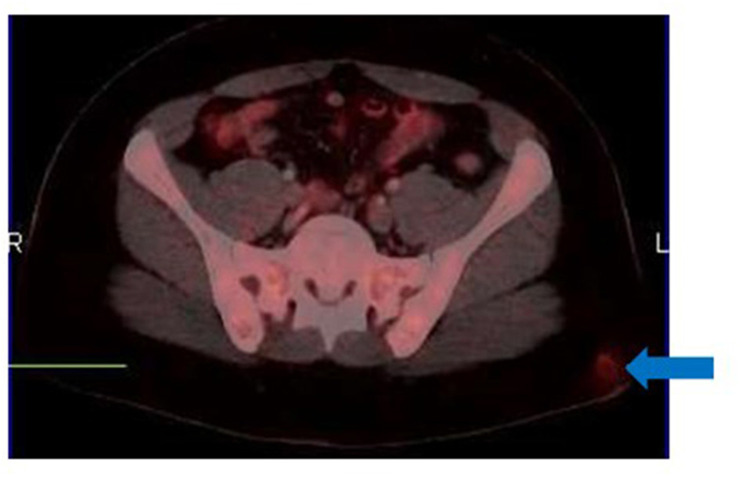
[18F]FDG PET/CT for suspected recurrent cutaneous melanoma. Fused trans-axial image shows a focus of uptake in the left gluteal region (arrow) mimicking subcutaneous involvement. There was no associated CT change. This focus corresponds to recent intramuscular injection site as confirmed from the clinical history.
